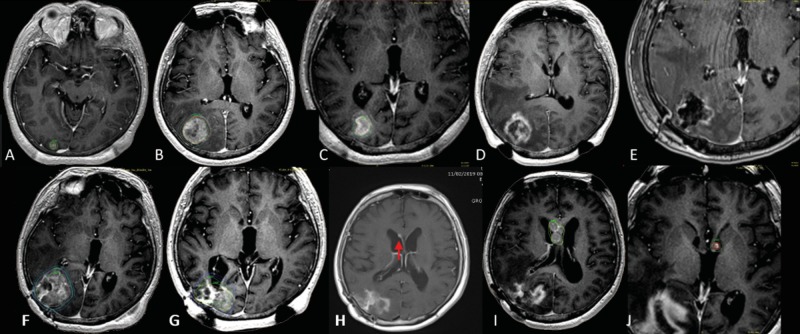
Two-session radiosurgery followed by surgery in a multiple metastases case in a lung cancer patient A. Initial plan showing a small occipital metastasis being treated with a single dose of 20 Gy to the 65% isodose line. B. Lesion progression eight months after initial treatment. Due to the size and volume (18.7 ml) of the lesion, the patient received a second course of two-session radiosurgery, at a dose of 15 Gy to the 46% isodose line. C. Thirty days later, the lesion volume was 1.3 ml, and the patient received a second session and third course of radiosurgery, at a dose of 11 Gy to the 50% isodose line. D Eight months later, 16 months after the initial treatment, the tumor had progressed, and the patient underwent surgery. E. Three-dimensional MRI after surgery, showing an apparent residual in the most medial aspect of the cavity; further radiation treatment was not prescribed. F. Four months after surgery, local tumor progression was observed, with the tumor volume being 38.2 ml, and the patient was administered a new course of two-session radiosurgery, consisting of a dose of 12 Gy to the 50% isodose line. G After 30 days, tumor response was adequate, with a tumor volume of 14.4 ml (62% reduction). The patient was administered the second session of radiosurgery, consisting of a dose of 12 Gy to the 50% isodose line. H. Eight months after the second course of two-session radiosurgery and 24 months after initial treatment, the occipital lesion appeared controlled, but a small intraventricular metastasis was observed (red arrow). I and J. Results of rescue radiosurgery at nine months, 25 months after initial treatment for intraventricular tumor dissemination.
